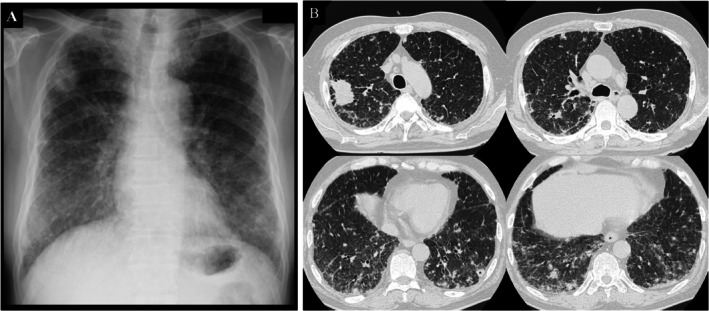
(A) Chest radiography performed on admission showed a mass shadow in the right upper lung field and small granular shadows in the bilateral lung fields. (B) Chest computed tomography also showed random distribution of granular shadows and nodules of 1–12 mm in size in both lungs, and nodule shadows with cavities in some of them. In addition, some infiltration shadows were shown under the pleura of both lower lobes. A 5‐cm large marginal irregular mass shadow was also observed in the upper right lobe.
